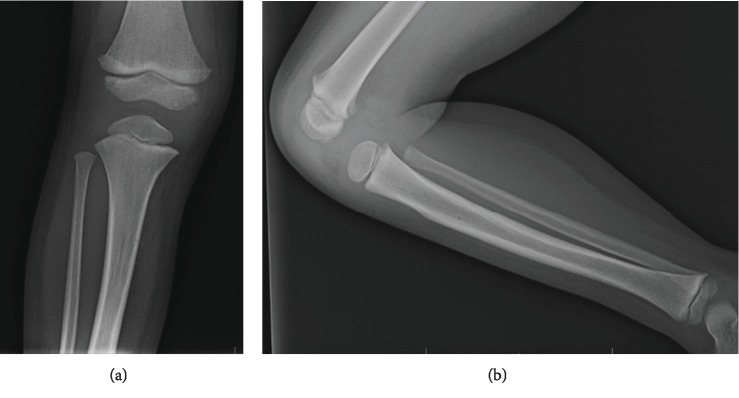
Anteroposterior (a) and lateral (b) plain radiographs of the patient at the time of discharge from initial hospitalization reveal no abnormalities aside from the small cortical defect made by the IO cannula in the proximal tibia.
